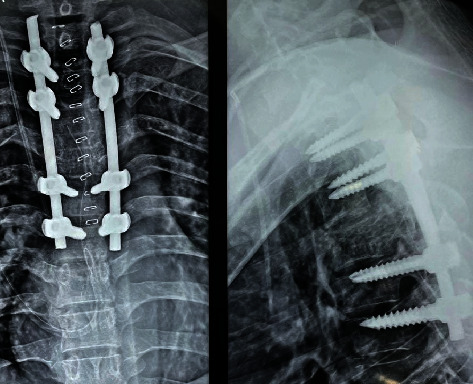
Postoperative X-ray showing posterior instrumentation and stabilization done T2 to T6 levels with pedicle screw fixation at T2, T3, T5, and T6.
